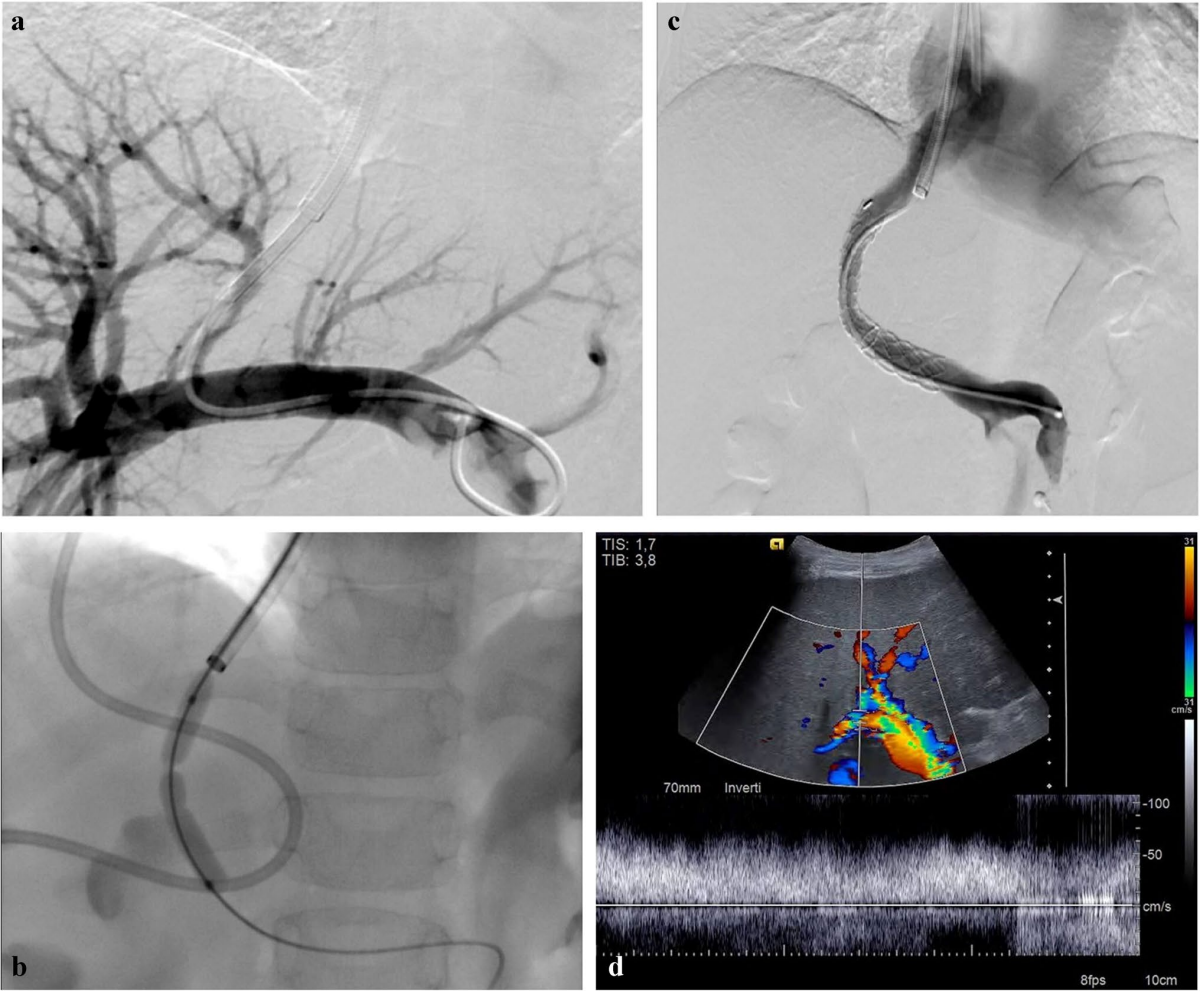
Transjugular intrahepatic portosystemic shunt (TIPS) placement in a 9-year-old girl with liver cirrhosis. a Posteroanterior (PA) portal venogram. b PA fluoroscopy image shows balloon dilation of the intrahepatic tract. Note the proximal and distal balloon notches indicating the length of the hepatic tract. c Portal PA venogram after stent positioning shows good flow through the TIPS. d Three-month follow-up axial Doppler US shows good patency of the TIPS with no late complications
